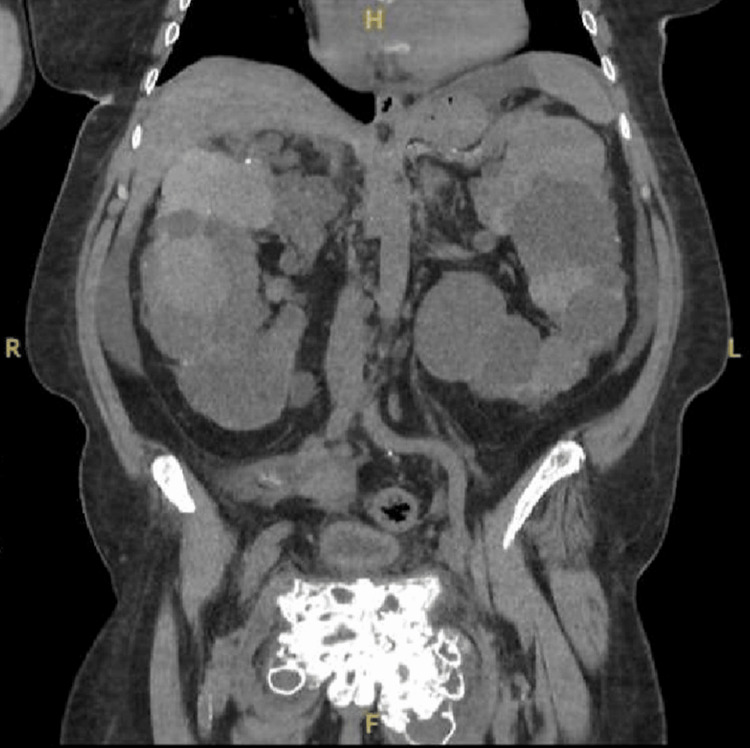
CT coronal view of the abdomen and pelvis demonstrates significant uremic tumoral calcinosis of the pubic symphysis, and polycystic kidneys are also shown.
